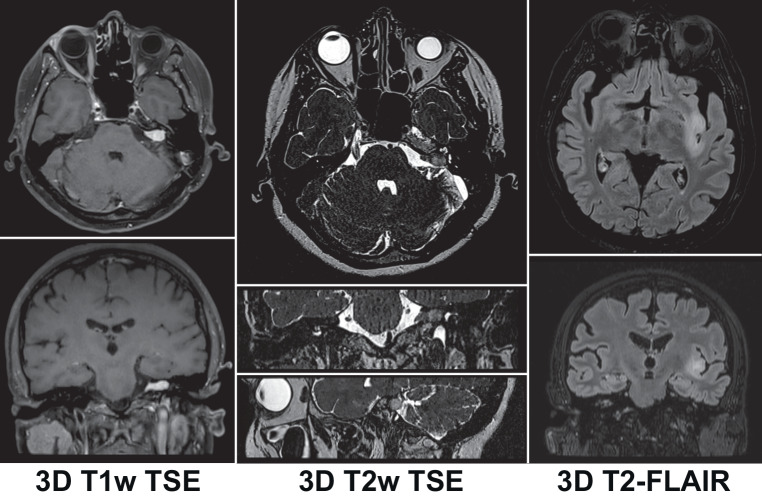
3D MRI sequences for intracranial SRT treatment planning. *Left*: 3D T1w TSE sequence in a patient with vestibular schwannoma. *Center*: 3D T2w TSE sequence in the same patient, *Right*: 3D T2-FLAIR sequence. Note: high-resolution multiplanar reconstruction
